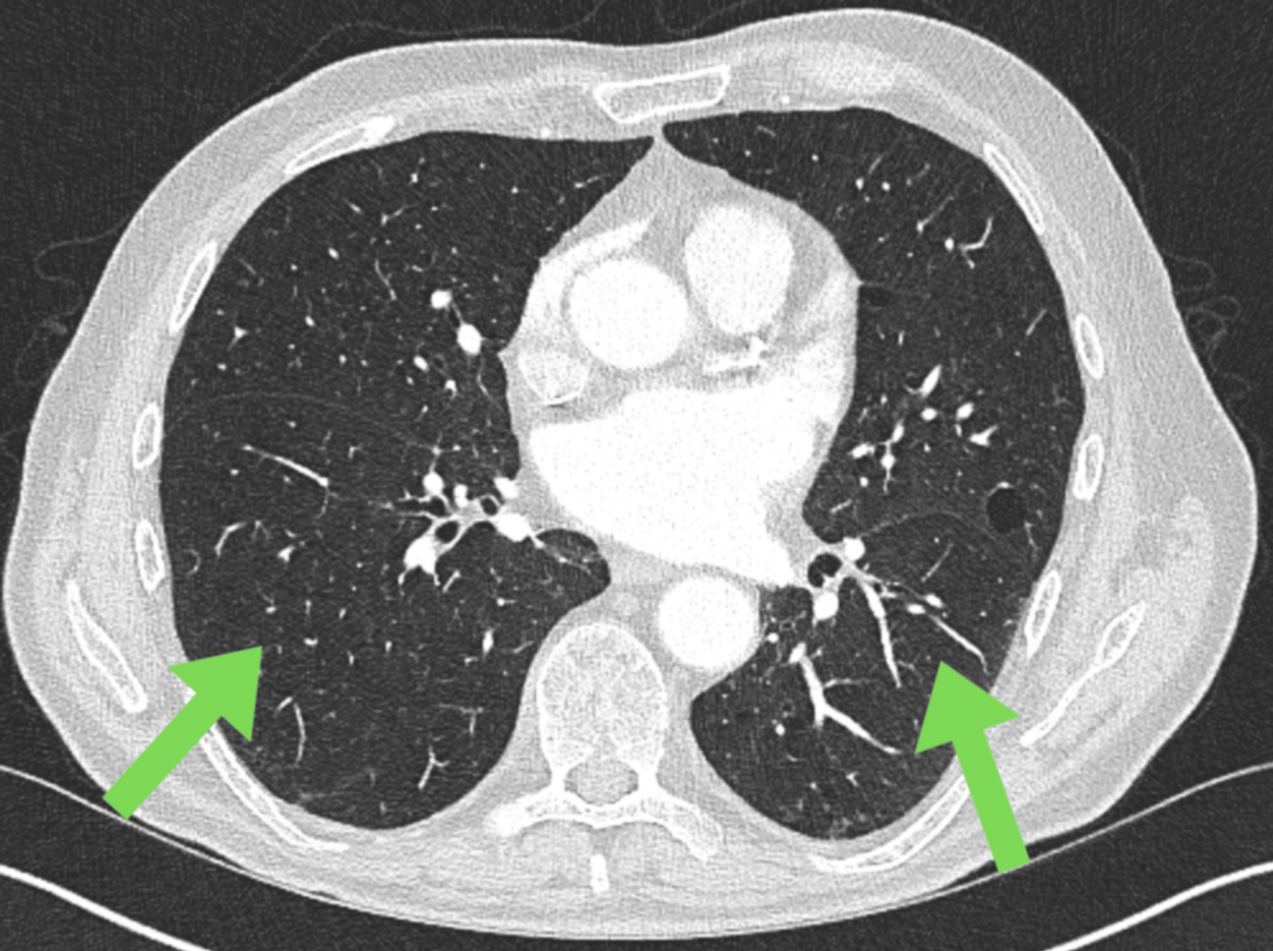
Clear CT thorax with resolution of previous findings Green arrows showing interval resolution of cavitatory and consolidatory lesions with resolution of effusion.
